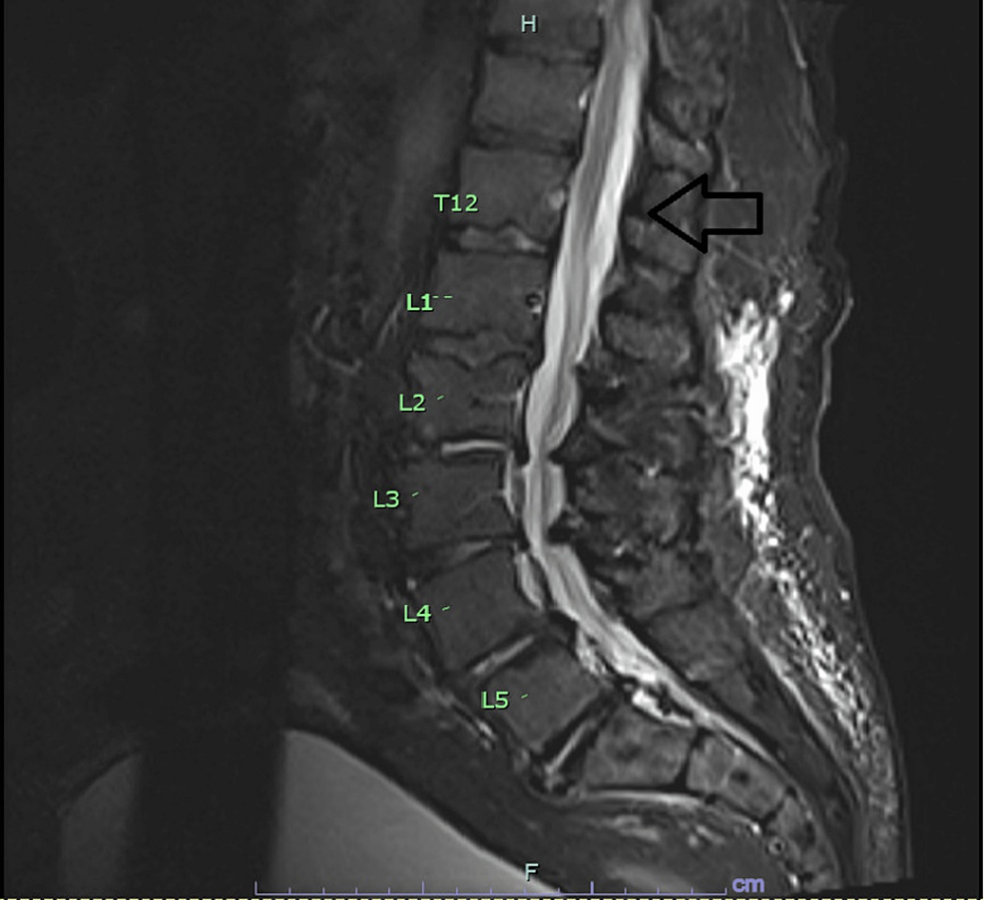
MRI of the lumbar spine showing epidural hematoma (arrow).
